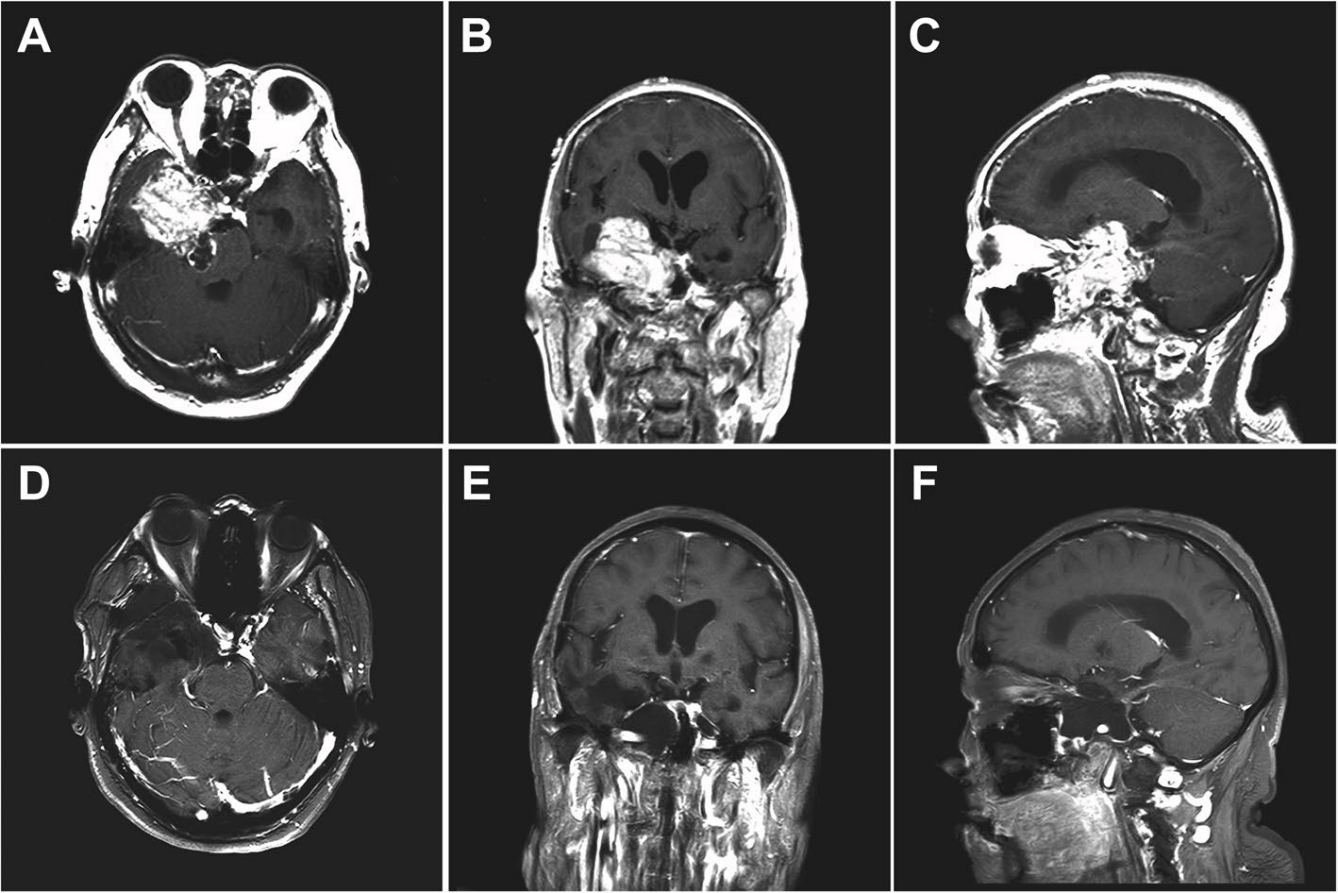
Right trigeminal schwannoma resection via right subtemporal keyhole approach. A–C Pre-operation MR images. D–F Postoperation MR images
